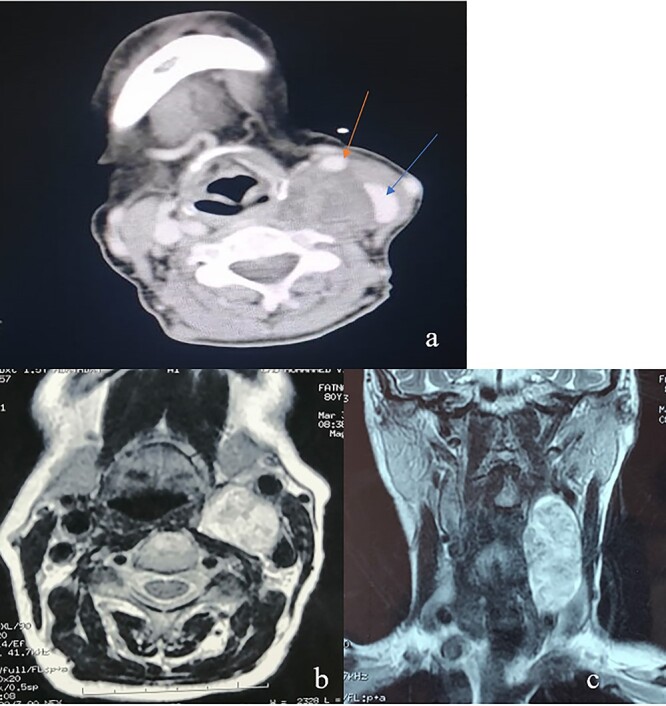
(a) An axial CT of the neck revealing an oval heterogeneous mass in the left carotid space measuring 37 × 30 × 57 mm; the IJV (blue arrow) is displaced laterally and the CCA (red arrow) is displaced anteriorly; this is often a characteristic sign of VNS; (b) an axial T2-weighted MRI; left carotid space mass, noninfiltrating widening the space between CCE and IJV; (c) an coronal T2-weighted MRI showing a left carotid space mass with vertical major axis.
